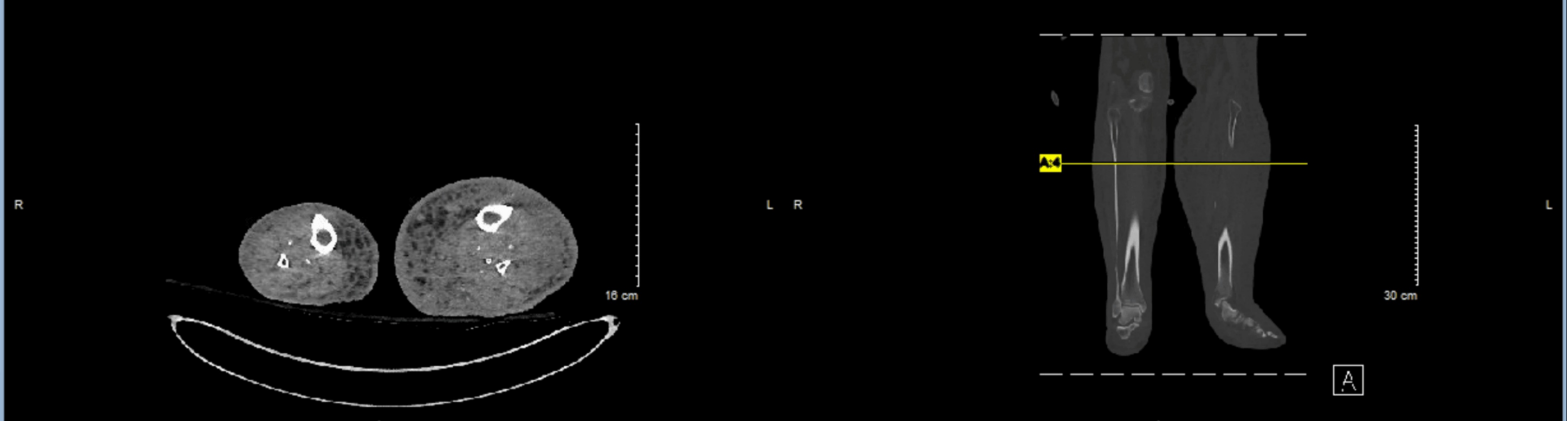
CT scan without contrast of bilateral extremities also showing greater soft tissue edema on the left compared with the right. CT: computed tomography
